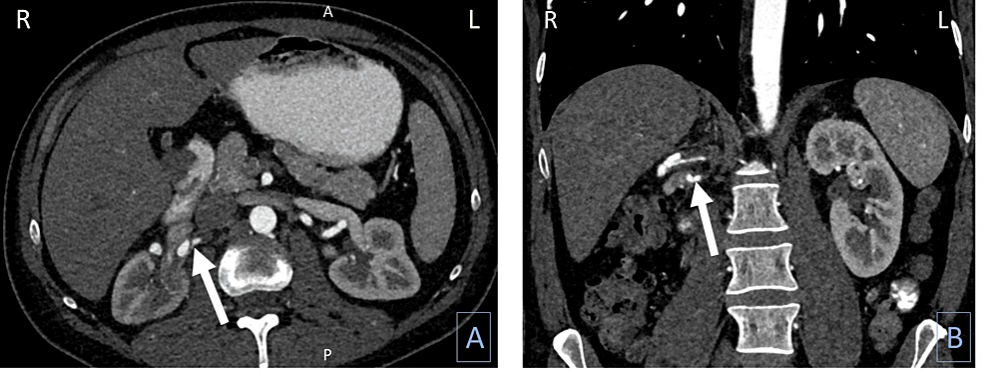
Repeat CT of abdomen/pelvis with IV contrast showing the dissection of the renal artery (arrow), (A) axial view and (B) coronal view. A: anterior; P: posterior; R: right; L: left.
